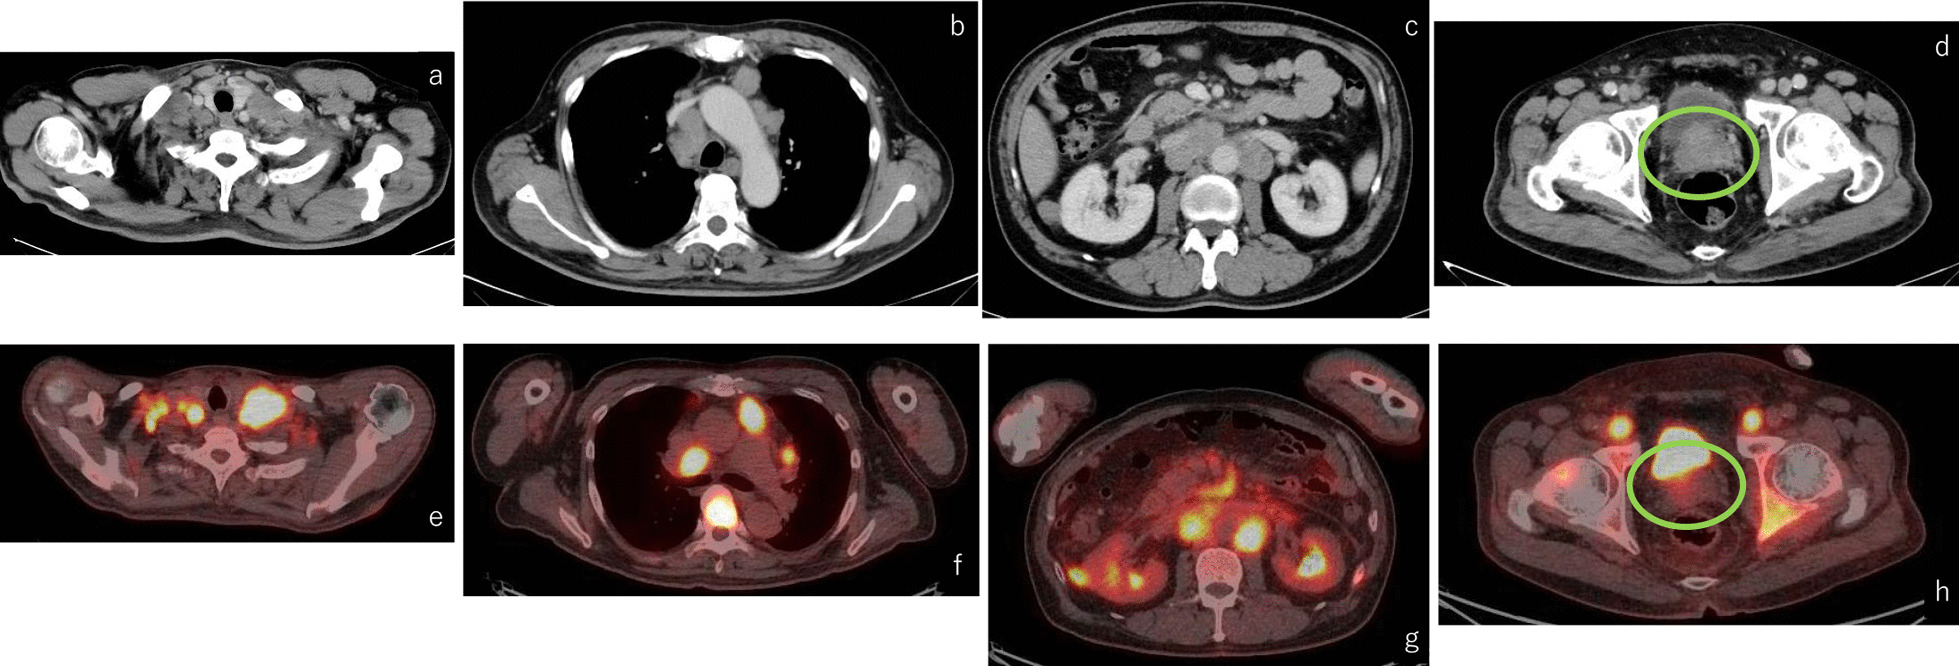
Computed tomography showing multiple swollen lymph nodes in both sides of his neck (a), mediastinum (b), abdomen (c), and pelvis (d). Positron emission tomography-computed tomography showing 18F-fluorodeoxyglucose accumulation in the same area and multiple bone metastases (e–h). Despite prostate enlargement, no FDG accumulation was observed (green circle)
